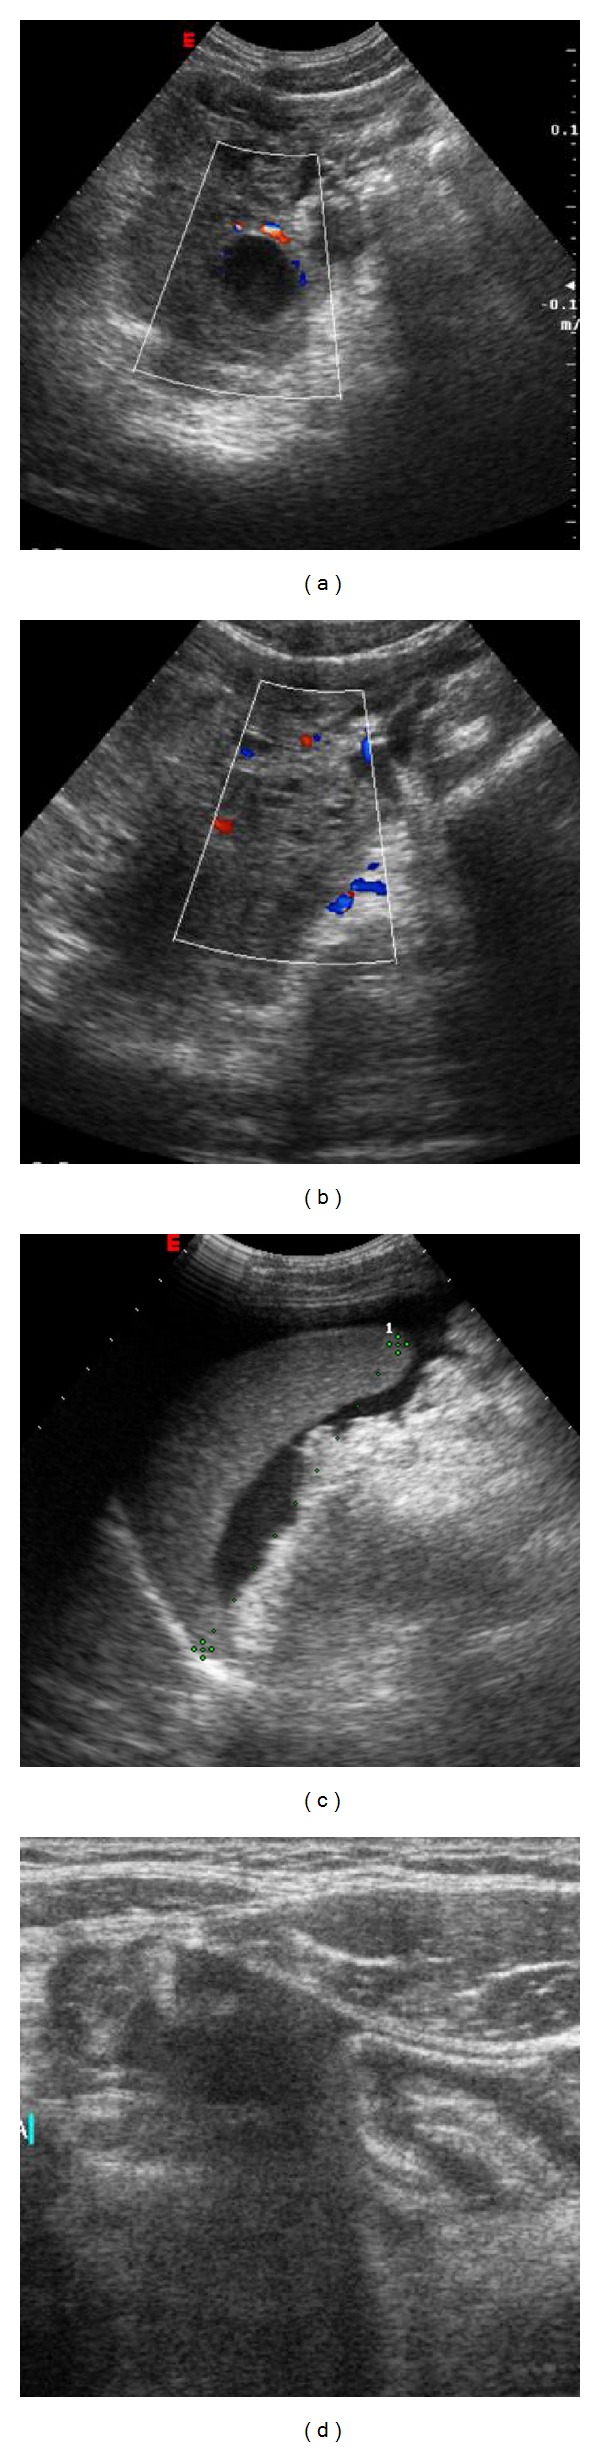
Transabdominal US shows on the right adnexal area a regular cyst with internal echoes and peripheral vascularization on Doppler evaluation (a). Free fluid into the pouch of Douglas (b) and around the spleen (c). (d) Ultrasonographic scanning with linear probe shows free fluid containing low level echoes in the right iliac fossa. No signs of appendicitis are appreciable.
